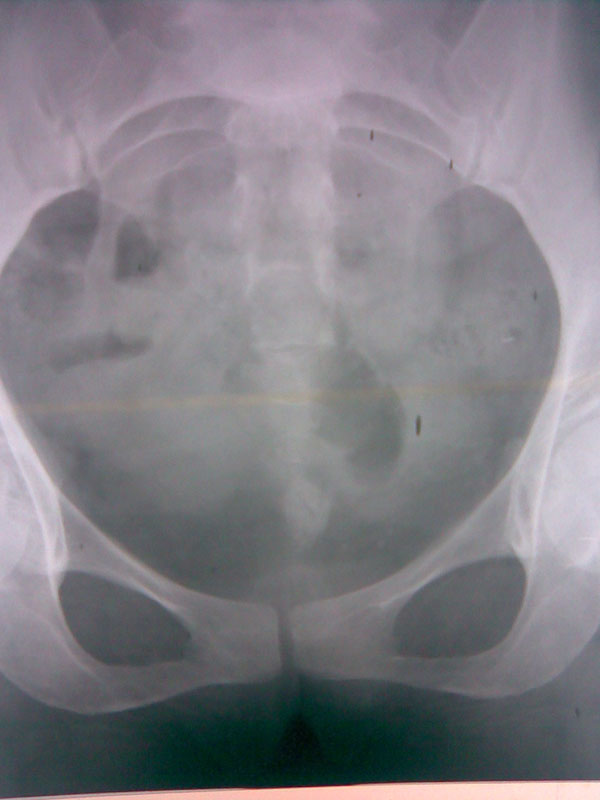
X-ray of the kidney, ureter and bladder showing radio-opaque irregular shadow in the posterior wall of the bladder.
